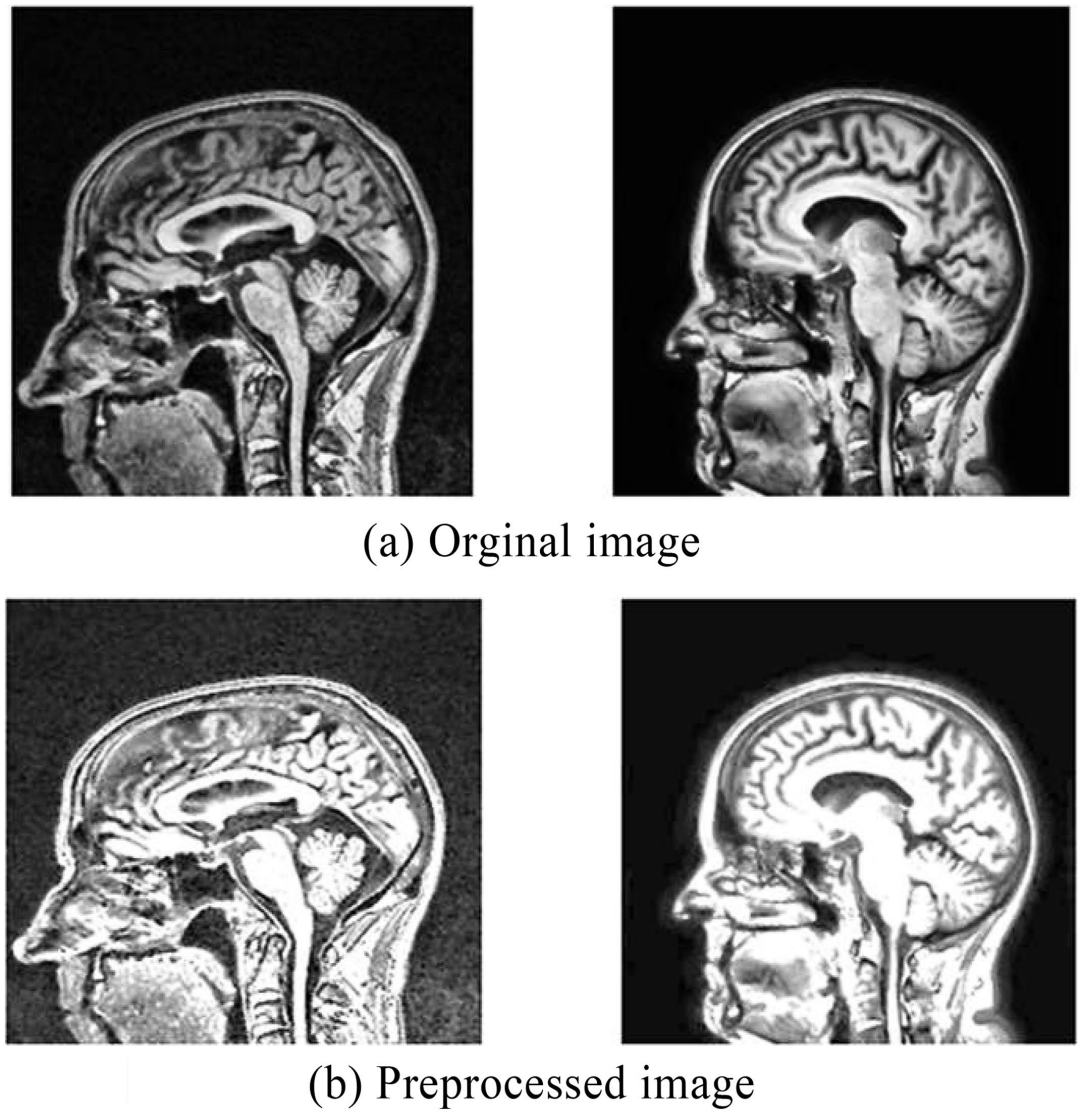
(a) Original MRI image before preprocessing. (b) Preprocessed MRI image.
